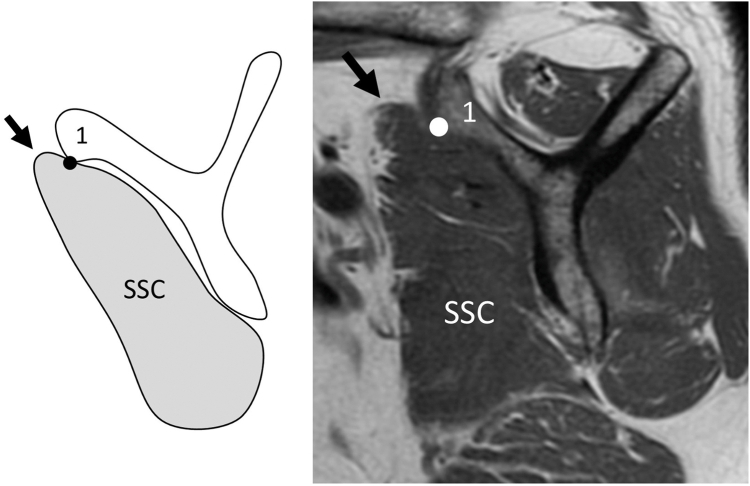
Subscapularis (SSC) atrophy, Grade 0 (Normal). The Y-shaped view was selected on T1-weighted oblique sagittal magnetic resonance imaging (MRI), defined as the slice visualizing both the base of the coracoid process and the lateral border of the scapular spine. In Grade 0, the superior margin of the SSC contour is located cranial to the anteroinferior edge of the coracoid base (*Point 1*).
